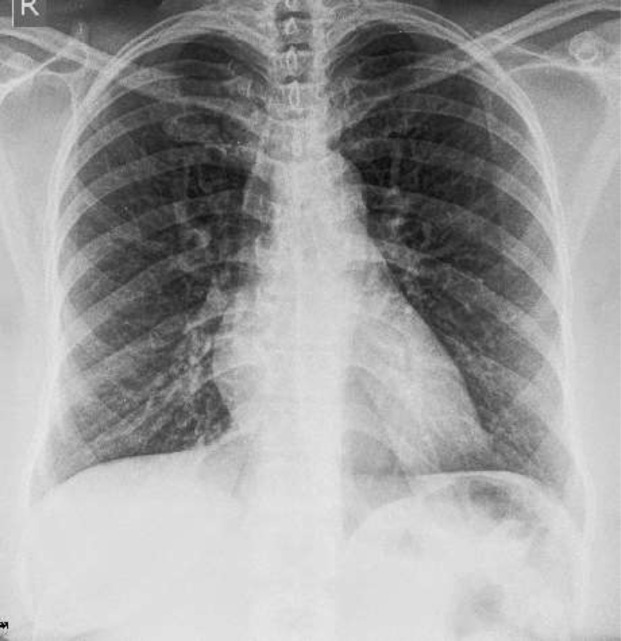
chest X-ray showing bilateral lower zone infiltrates
